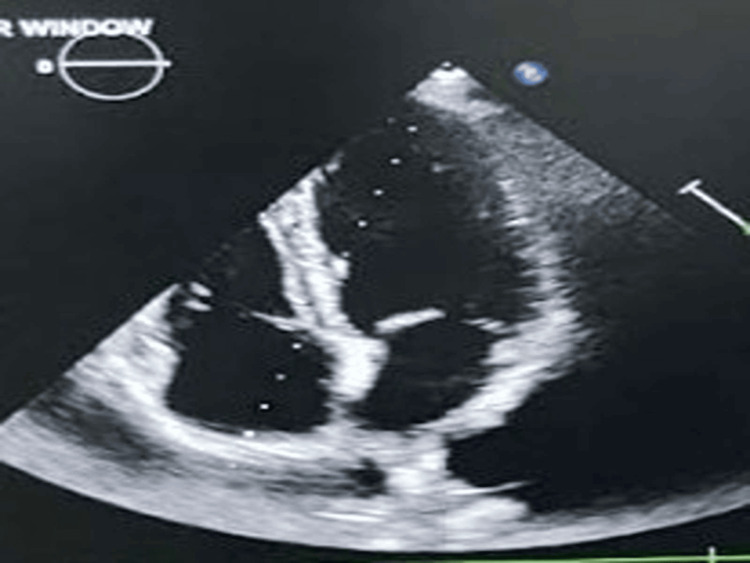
Apical four-chamber view showing complete resolution of the ball valve thrombus with anticoagulation.
